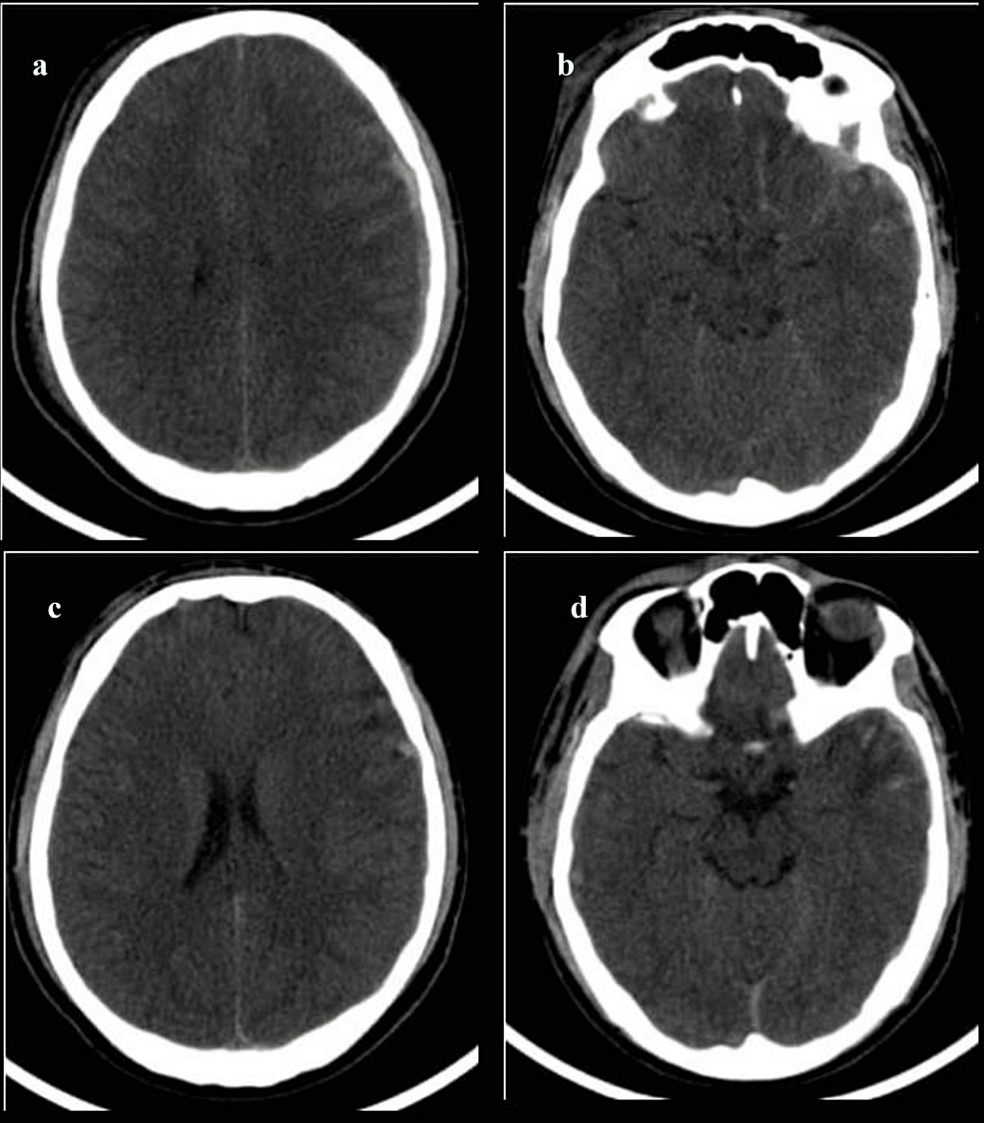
CT of a 21-year-old male patient following road traffic accident Initial admission CTs show (a) subdural hemorrhage in the left frontoparietal convexity with effacement of basal cisterns and (b) traumatic subarachnoid hemorrhage - Rotterdam score 4. Sequential CTs after 24 hours show (c and d) significant reduction in subdural hemorrhage resolution of the basal cisternal effacement, however, subarachnoid hemorrhage persisted - Rotterdam score 3.
